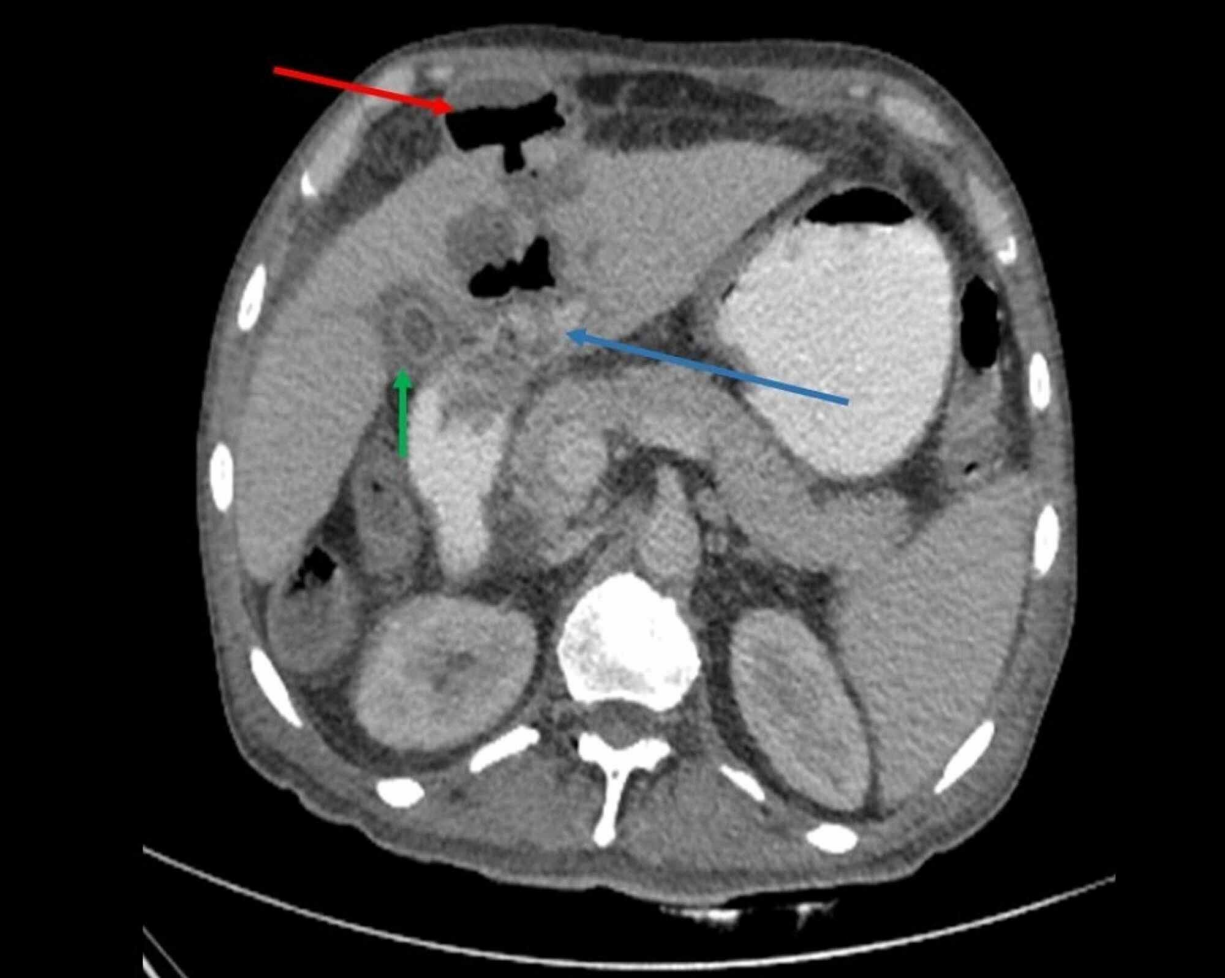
Axial contrast-enhanced CT abdomen showing extravasation of oral contrast into liver abscess (blue arrow). Note the reactive edematous thickening of gall bladder (green arrow) and pre-peritoneal rupture of abscess (red arrow).
